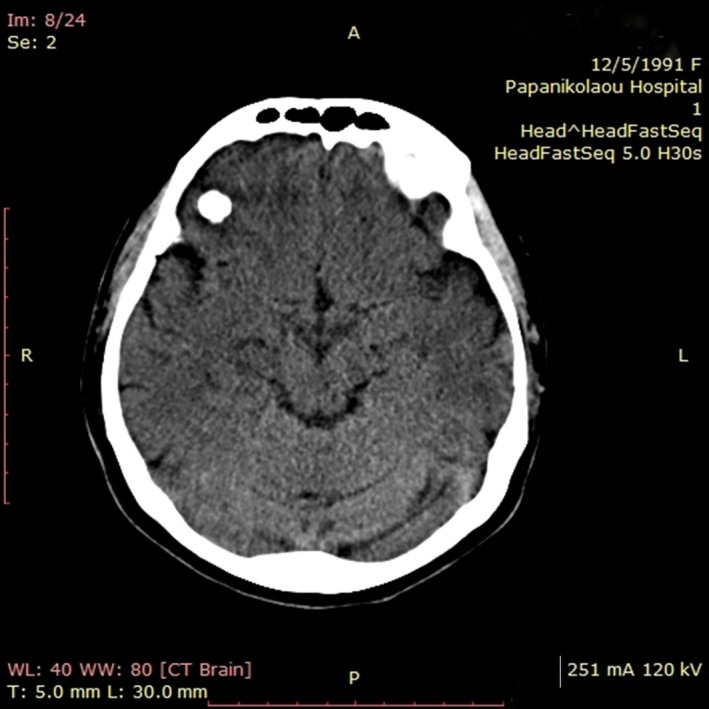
Axial brain CT scan depicting a bone density mass in the right frontal lobe region
